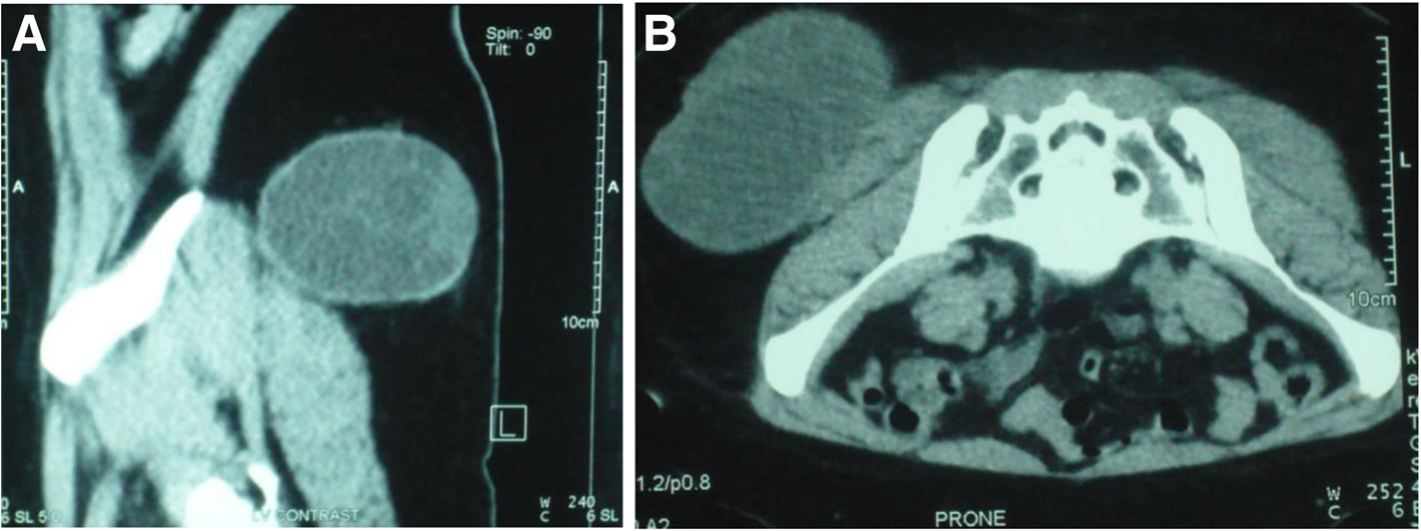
(A and B) Computed tomography; Parasagittal and axial view of the pelvis showing a cystic mass involving the right gluteus medius measuring 5x6cm with multiple daughter cysts inside the parent lesion.
